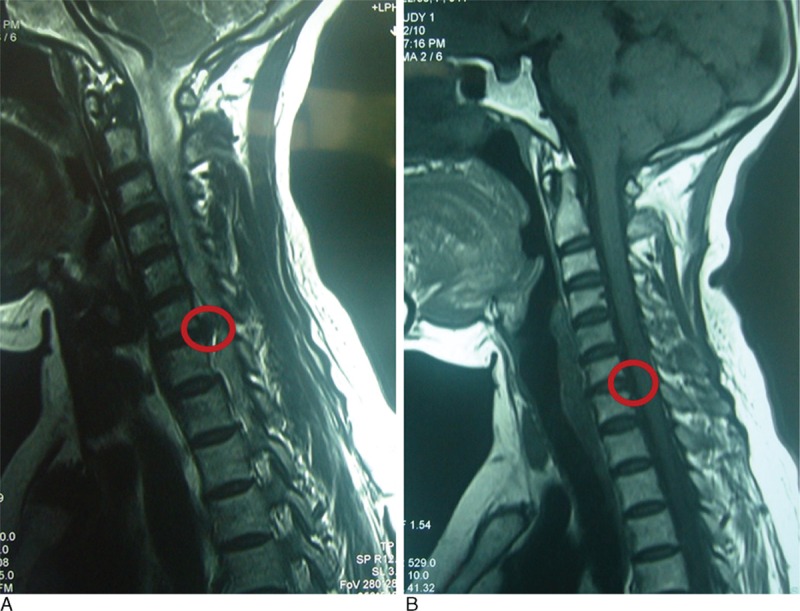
(A) Protrusion of the C6–7 disc demonstrated via a preoperative magnetic resonance imaging (MRI) scan. (B) Reduced protrusion of the C6–7 disc demonstrated via a postoperative MRI scan.
